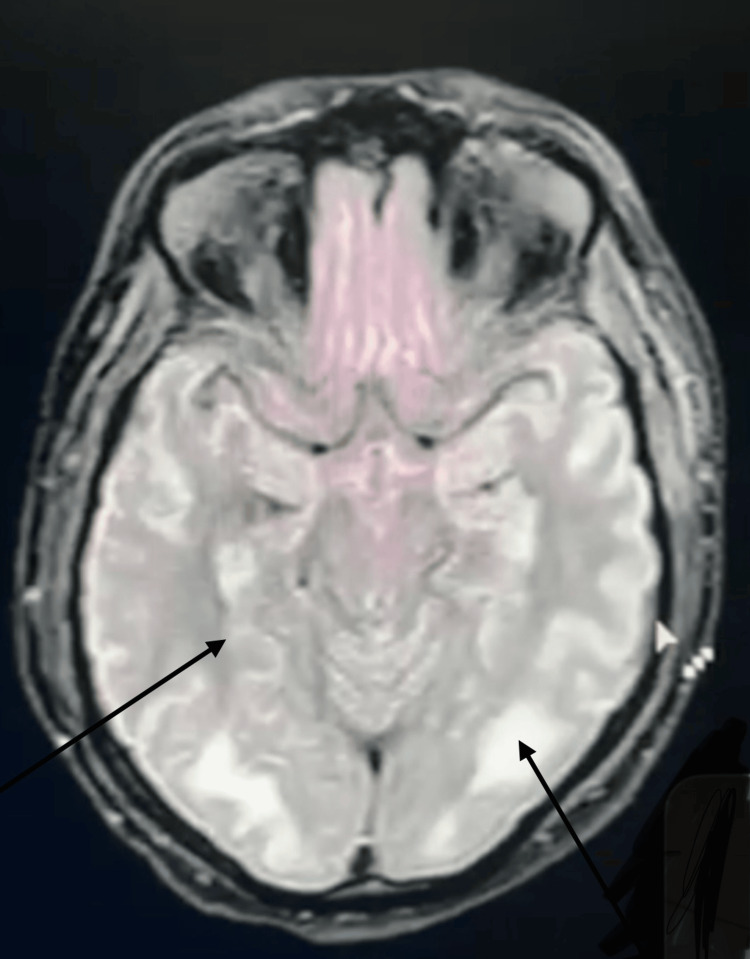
Axial T2-weighted brain MRI obtained on day three of hospitalization (10 days after symptom onset) showing a trident-shaped hyperintensity in the central pontine region, consistent with central pontine myelinolysis (CPM). Diffuse white matter hyperintensities suggest associated demyelination. No gadolinium contrast was administered
